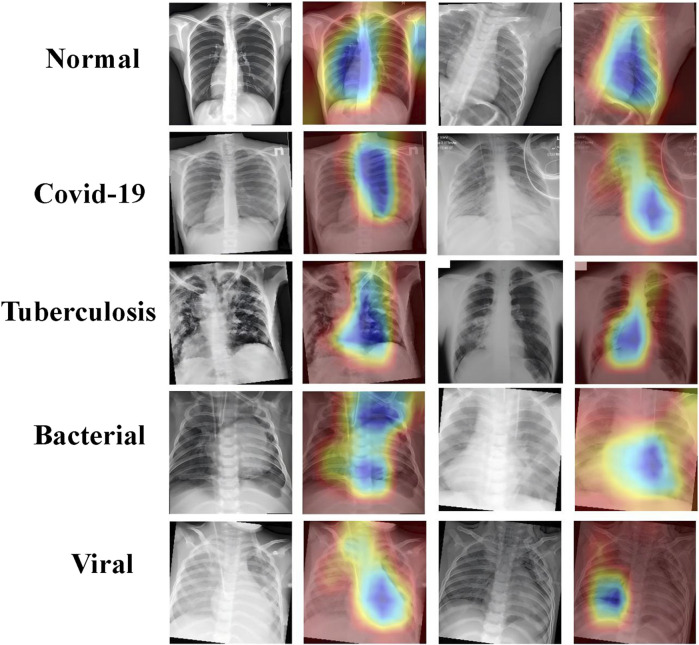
Grad-CAM visualizations highlighting key regions in chest X-rays for normal, COVID-19, tuberculosis, bacterial, and viral pneumonia.
